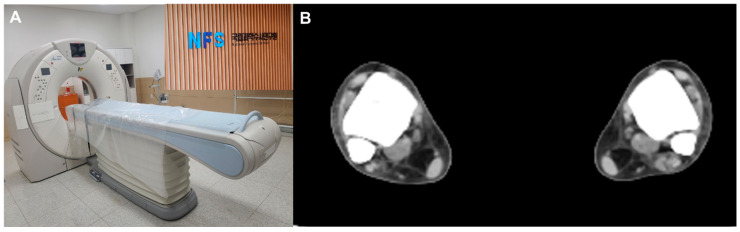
(A) All subjects were screened postmortem computed tomography, (B) A single axial image 1 cm above the tibial plafond, a level where the length of the distal anterior tibial tubercle is the greatest, was selected for measurement.
